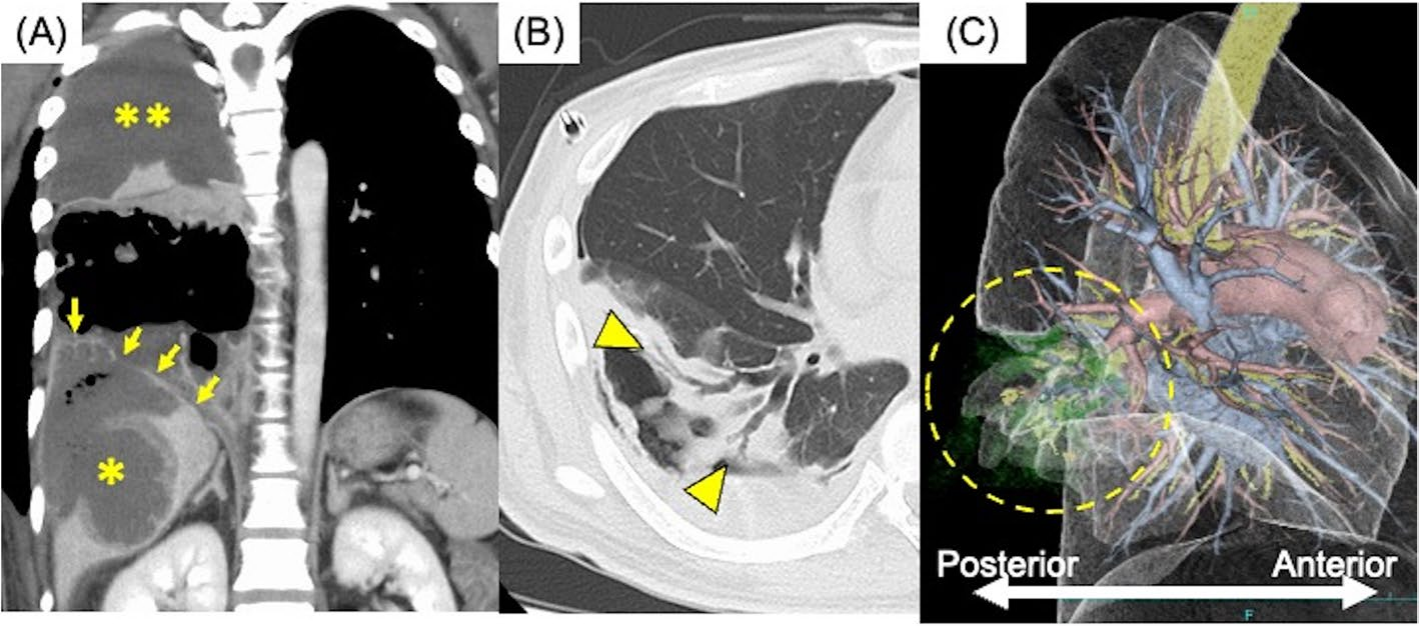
Computed tomography (CT) showed (A) an amebic abscess of the liver (yellow single asterisk) and amebic pleuropulmonary empyema (yellow double asterisk), although the diaphragm was intact (yellow arrows). B A bronchial fistula was identified in the right basal bronchus (yellow arrowheads). C Three-dimensional CT revealed a broad defect of the right lower lobe due to amebic infection (yellow dotted circle)
